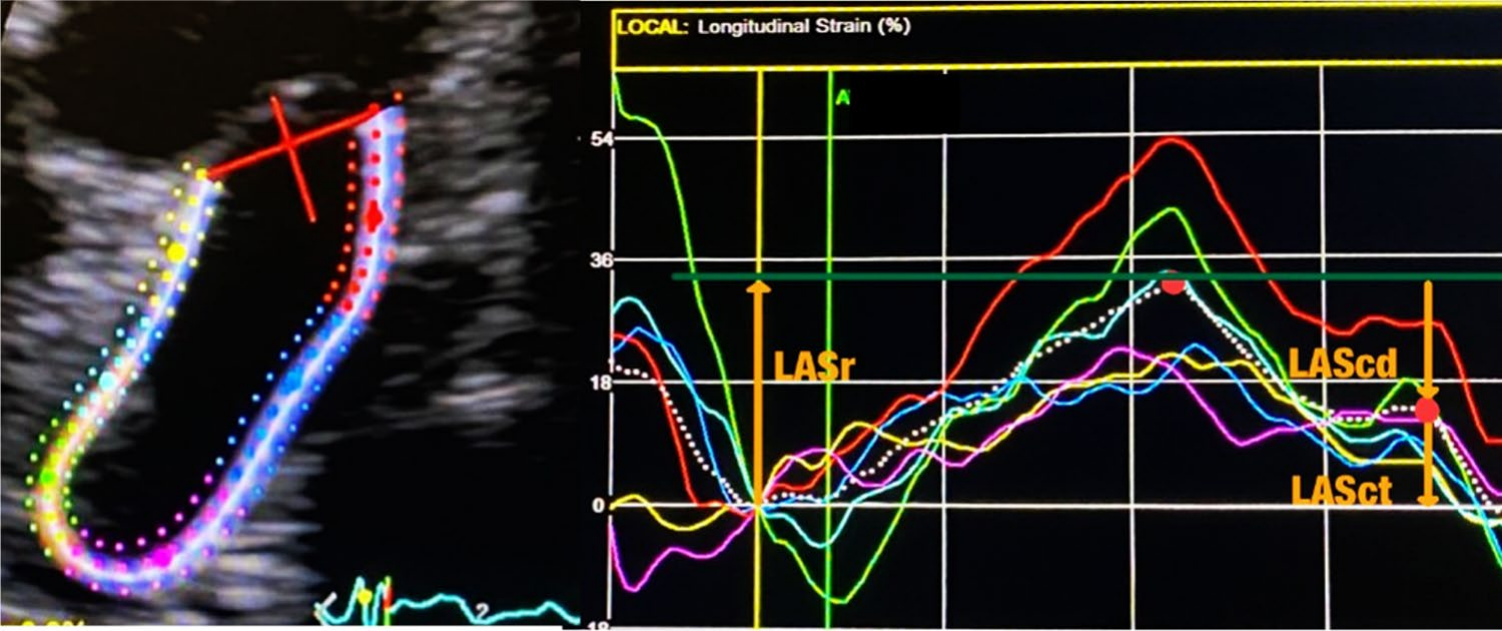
Atrial segmentation for longitudinal strain quantification using 2D echocardiography
